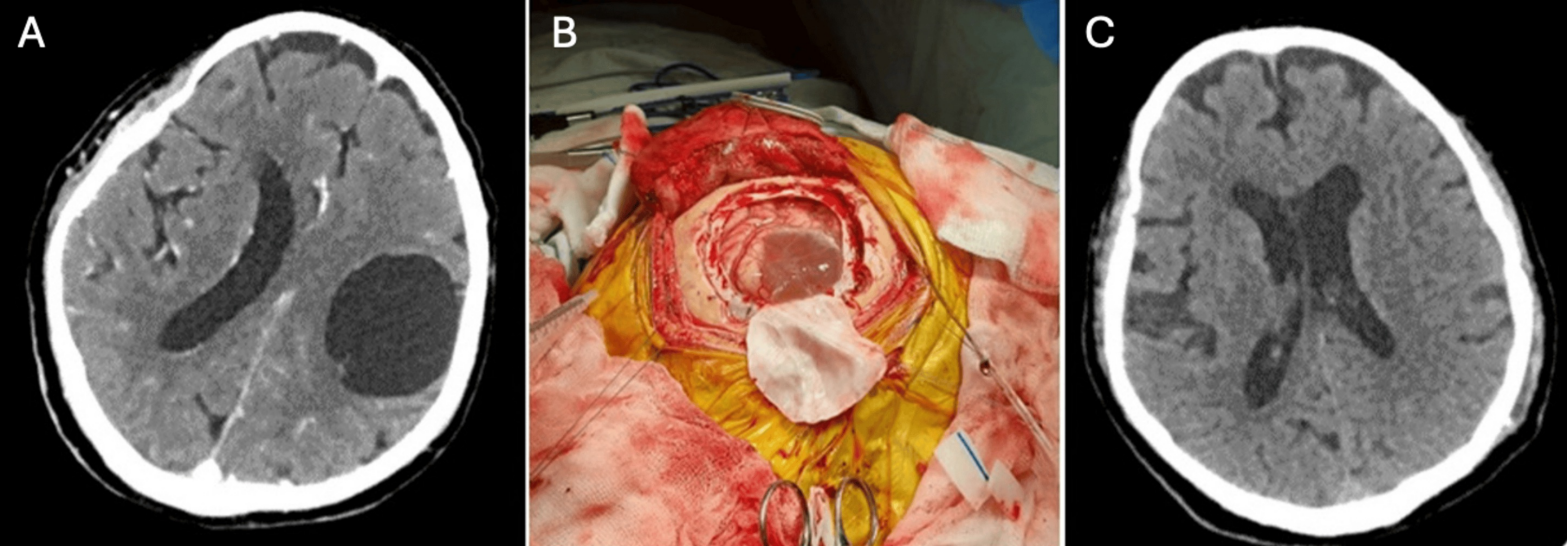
CT scan of the head shows a giant cysticercus cyst in the left frontoparietal region (A). Intraoperative image showing a cysticercosis cyst over the cerebral cortex (B). Control CT after surgery shows complete resection of the cysticercosis without complications (C) CT: computed tomography
